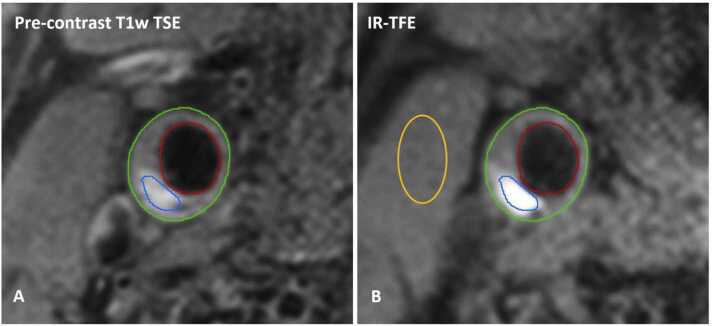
Carotid CMR images of an IPH-positive plaque. (A) The lumen (red) and outer vessel wall (green) were delineated on the pre-contrast T_1_w TSE image and used to determine the total wall volume. (B) Intraplaque hemorrhage (IPH) was delineated based on hyperintense signal in the bulk of the plaque on the 3D-T_1_w inversion recovery turbo field echo images. The IPH signal intensity ratio was defined as the maximum signal intensity within the IPH region (blue contour) divided by the average signal intensity of the adjacent muscle tissue (orange contour). *3D* three-dimensional, *IR-TFE* inversion recovery turbo field echo, *CMR* cardiovascular magnetic resonance, *T1w* T1-weighted, *TSE* turbo spin echo
